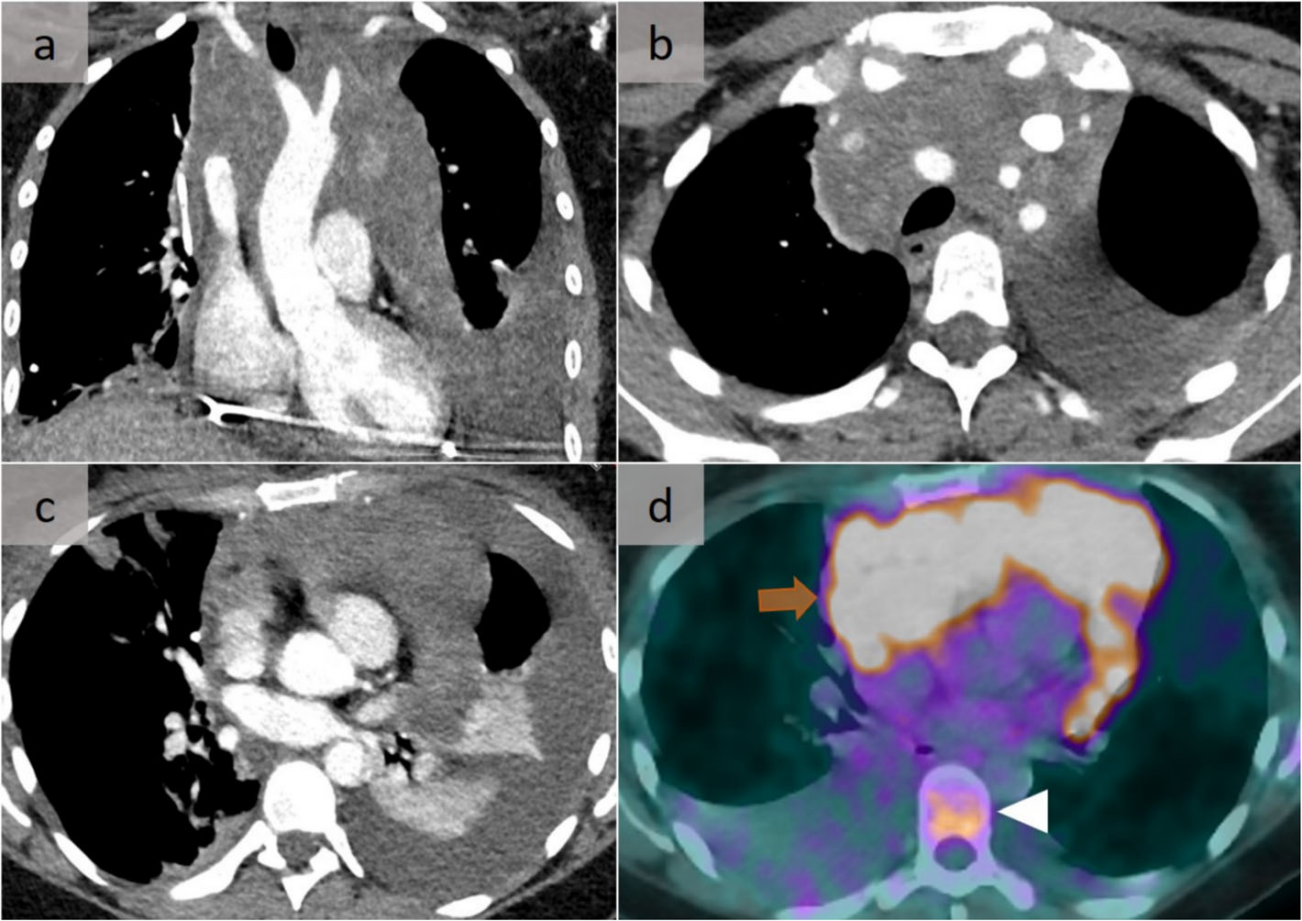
Hodgkin’s lymphoma. Diffuse mediastinal involvement well demonstrated on a coronal (a) and two successive axial slices (a, b). There is a contiguous pericardial involvement (c), with increased metabolic activity on the 18F-FDG PET-CT (d) (arrow). Note also the increased metabolic activity of the D11 vertebrae, suspicious for bone involvement (arrowhead), the partial resolution of the left pleural effusion following drainage, as well as a new right pleural effusion
